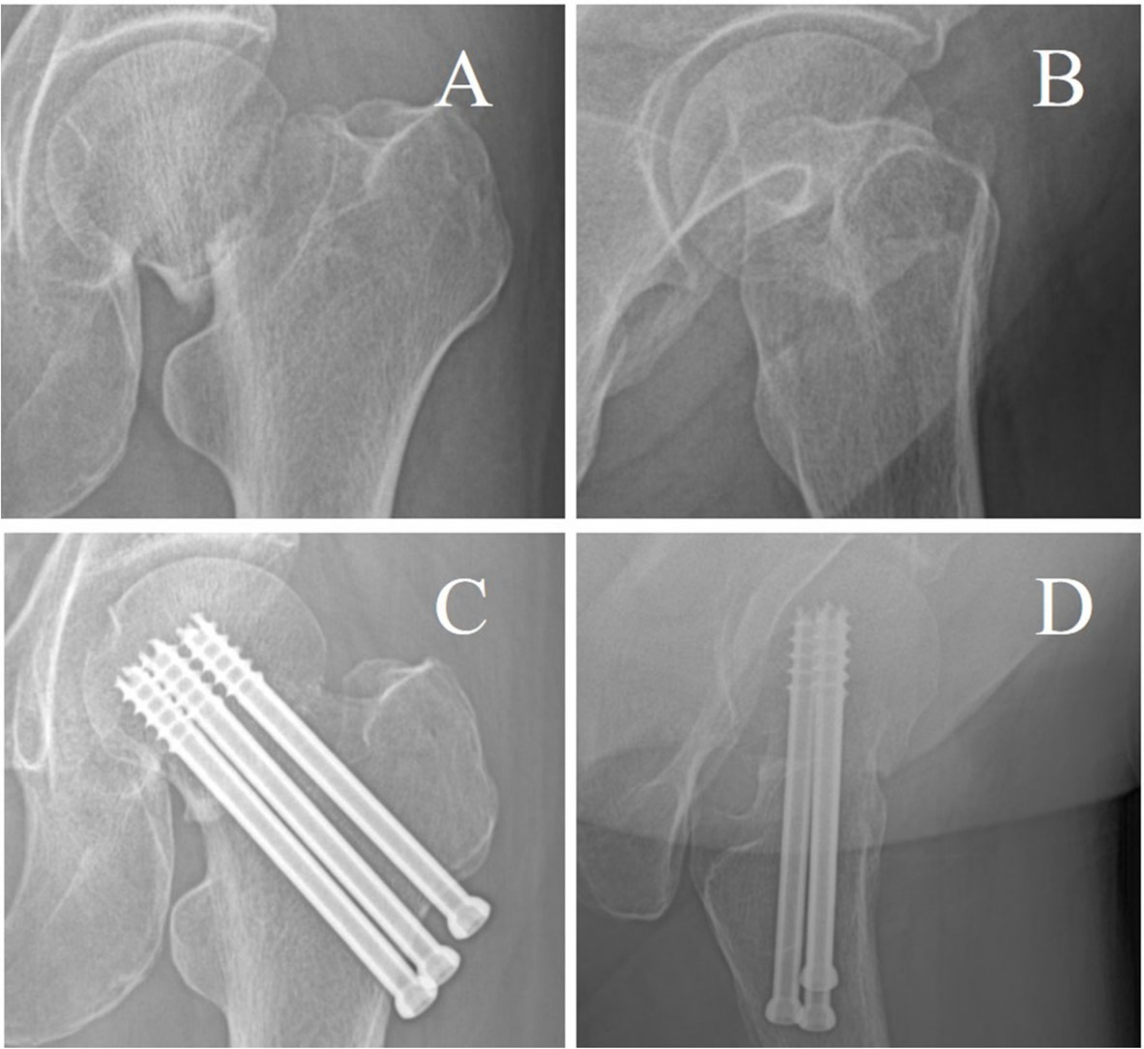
Typical case of three hollow screws for femoral neck fractures. a: Preoperative anteroposterior X-ray radiography b: Preoperative lateral X-ray radiography c: Postoperative anteroposterior X-ray radiography d: Postoperative lateral X-ray radiography
